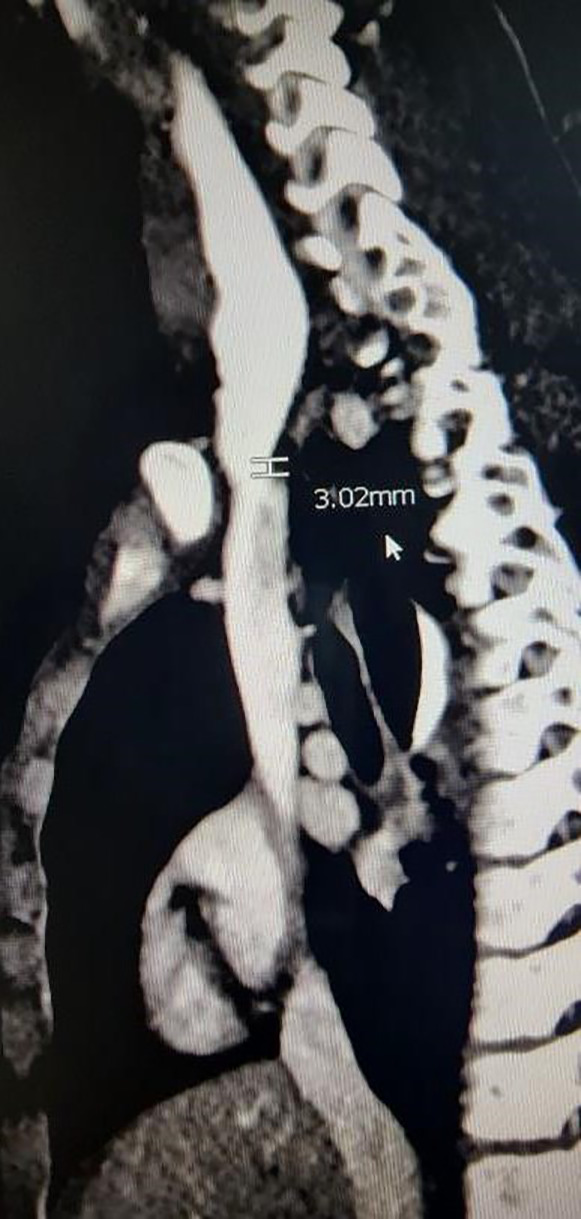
CT chest confirming a short-segment esophageal stricture.
